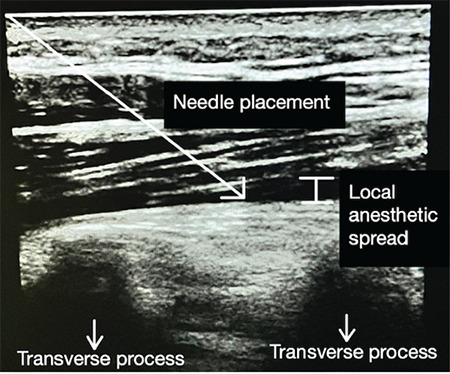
The USG image of the erector spinae plane block USG, ultrasonography.
